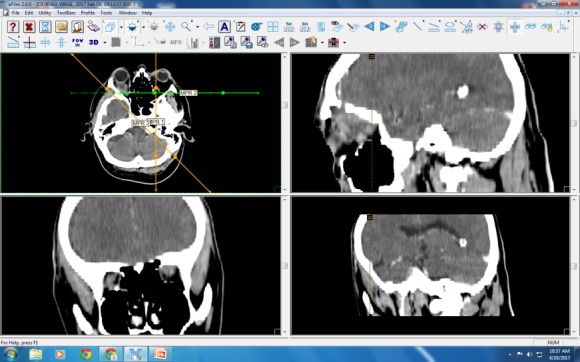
Craniocerebral CT – intraorbital lesions with frontal sinus invasion
